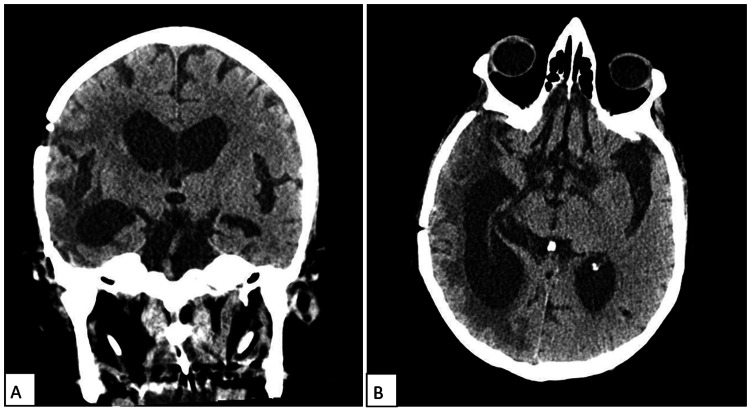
Postoperative CT The coronal (A) and axial (B) views show right pterional craniotomy and right cerebral hemispheric hypodensity with right lateral ventricle ex vacuo phenomenon.
